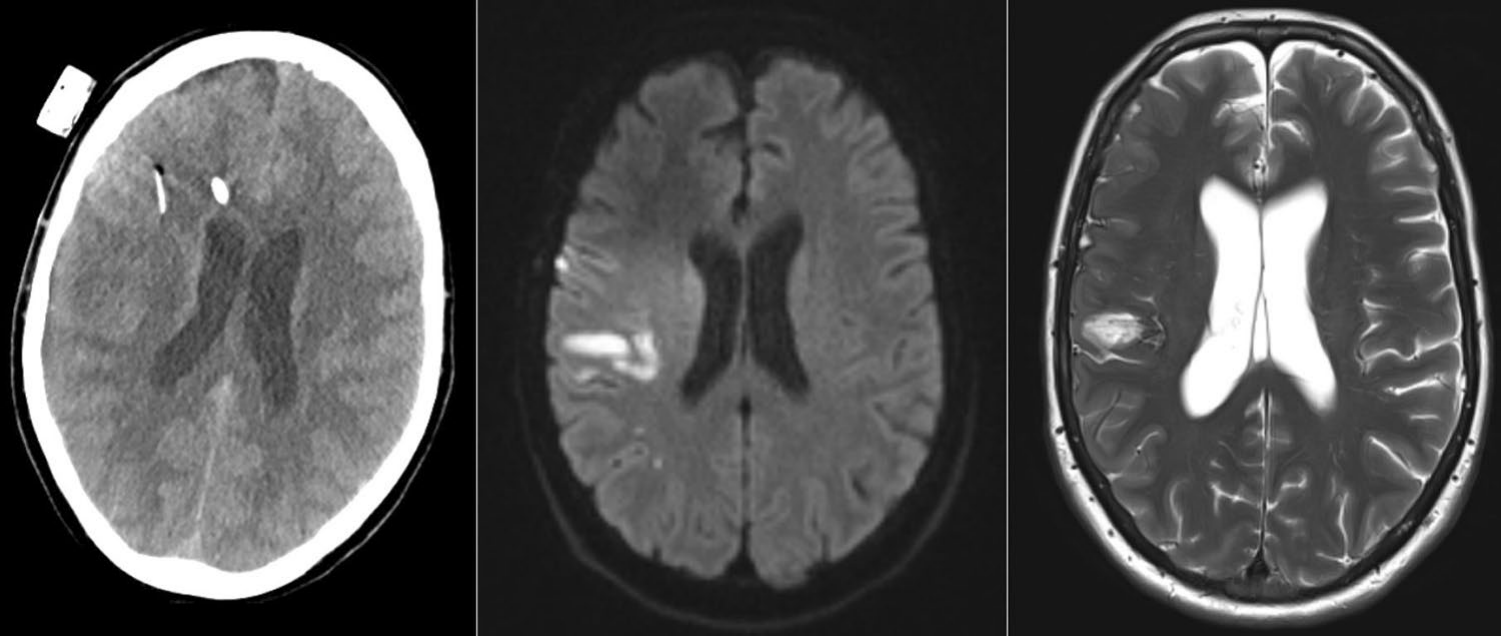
Infarction outside the vascular territory of the probe in patient 4. The patient suffered from aneurysmal subarachnoid hemorrhage with subsequent severe vasospasms causing an ischemic infarction. While the probe was inserted into the anterior MCA territory (left, brain CT without contrast), an ischemic infarction developed in the central MCA territory and was visible on initial MR scan (middle, diffusion-weighted imaging) and on follow-up MR imaging (right, T2-weighted image)
